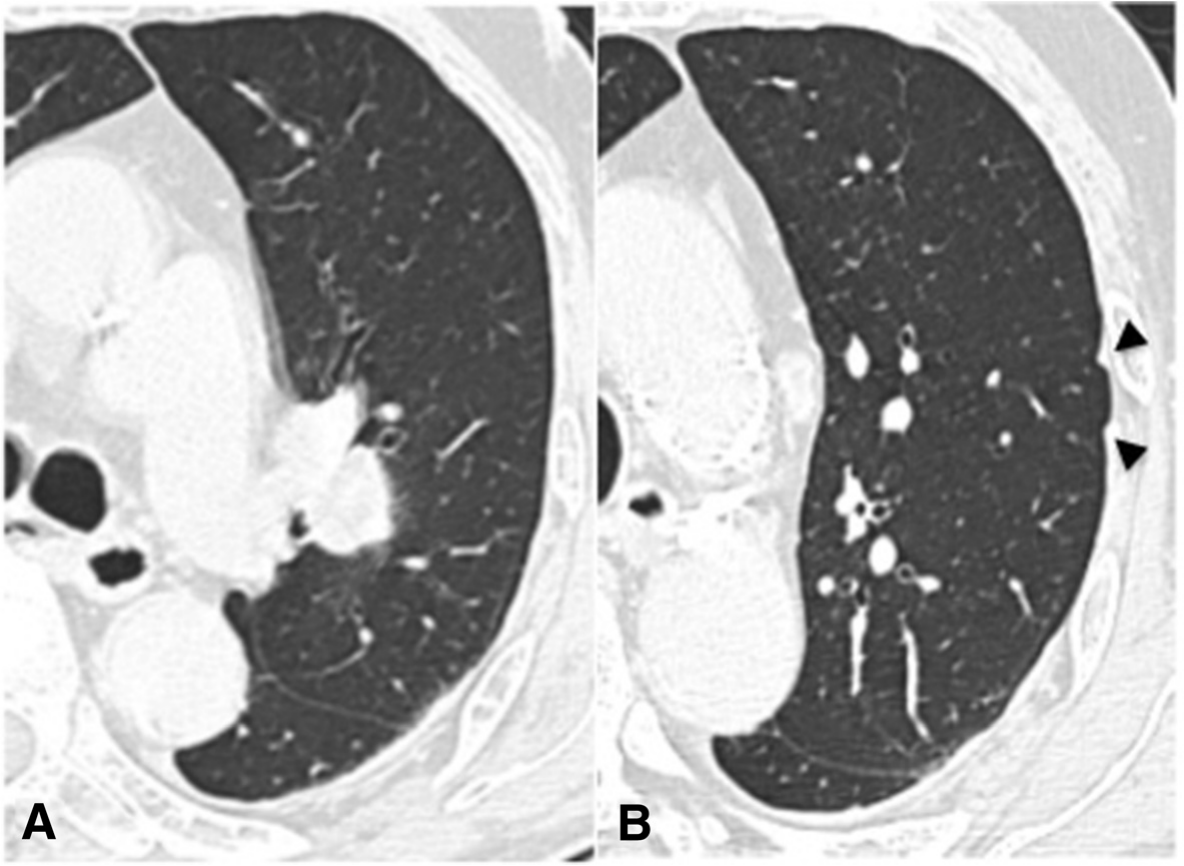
Computed tomography. (A) Computed tomography demonstrated a pulmonary nodule (23 mm in diameter) in the left hilum. (B) Two nodules in the subpleural space (7 and 9 mm in diameter, respectively) were also shown, but not identified before surgery (arrowheads).
